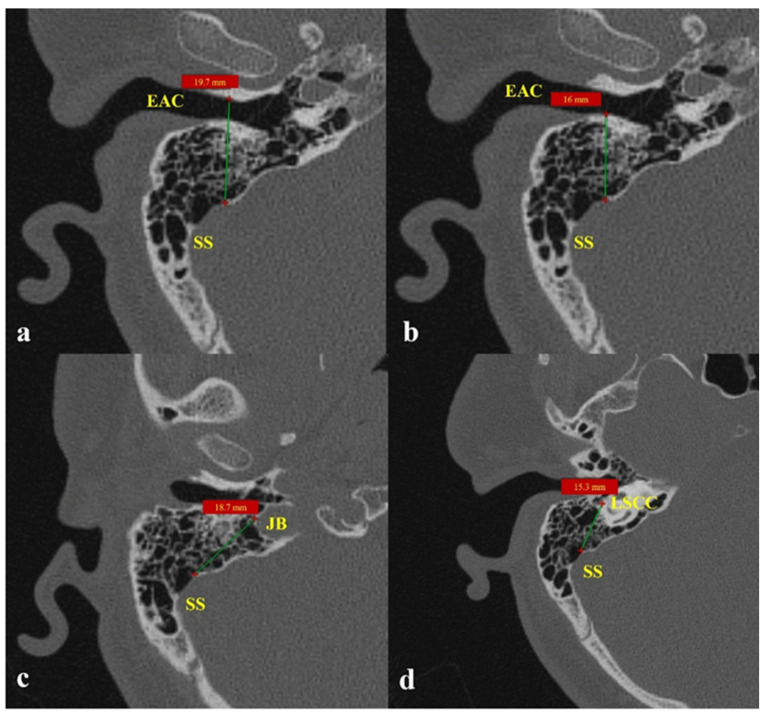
Distances from sigmoid sinus dehiscence (SSD) to adjacent key anatomical structures on axial CBCT images. (a) Shortest distance from SSD to the anterior wall of the external auditory canal (EAC); (b) Shortest distance from SSD to the posterior wall of the EAC; (c) Shortest distance from SSD to the jugular bulb (JB); (d) Shortest distance from SSD to the inferior border of the LSCC.
